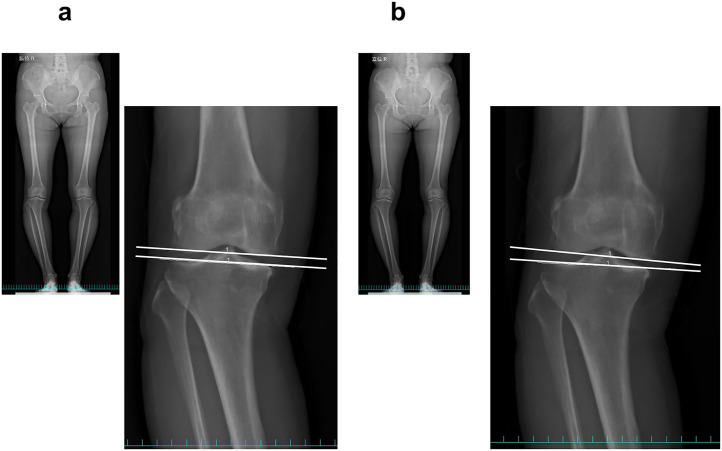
The representative alignment of the joint line convergence angle formed by two white lines, whole-leg radiographs in nonweight-bearing (a) and weight-bearing (b).
